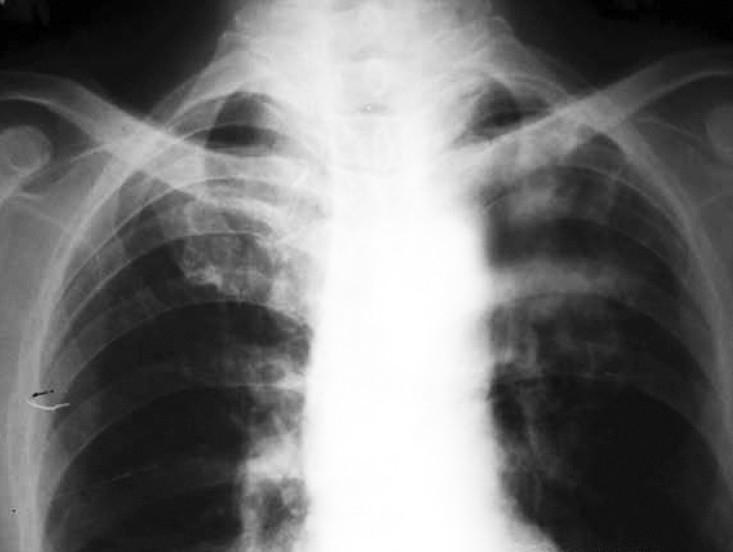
X-ray chest PA view post-treatment showing improvement
